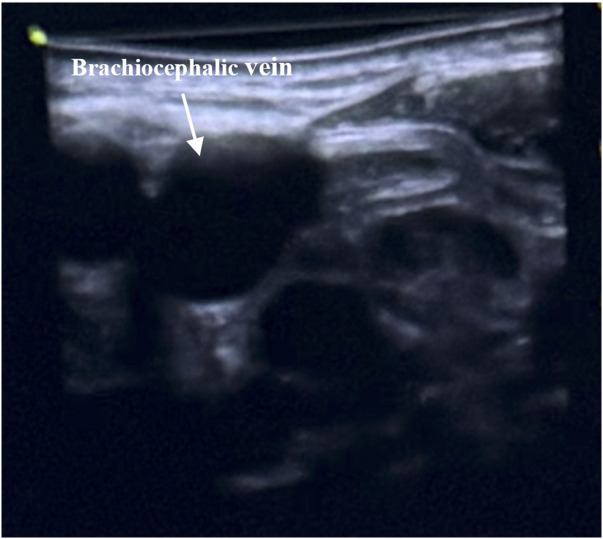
Ultrasound-guided venous cannulation.
